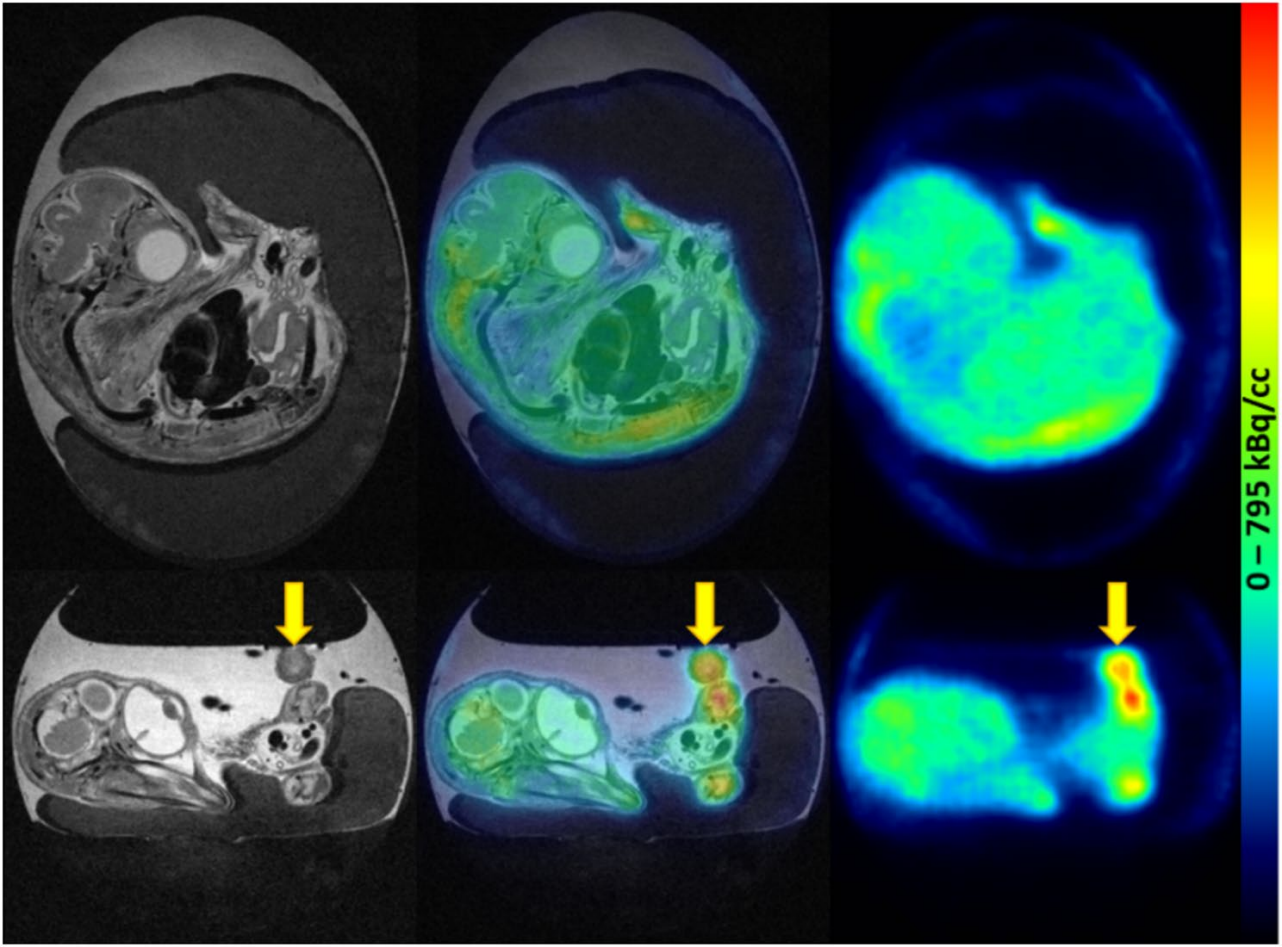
Representative images of [^18^F]FDG distribution in the fertilized chicken egg on EDD18. PET scan was started 60 min p.i. of 5.31 MBq [^18^F]FDG. Left: T2-weighted TurboRARE images, right: PET images, middle: fused PET/MR images. The xenograft (HT29) is marked with yellow arrows
